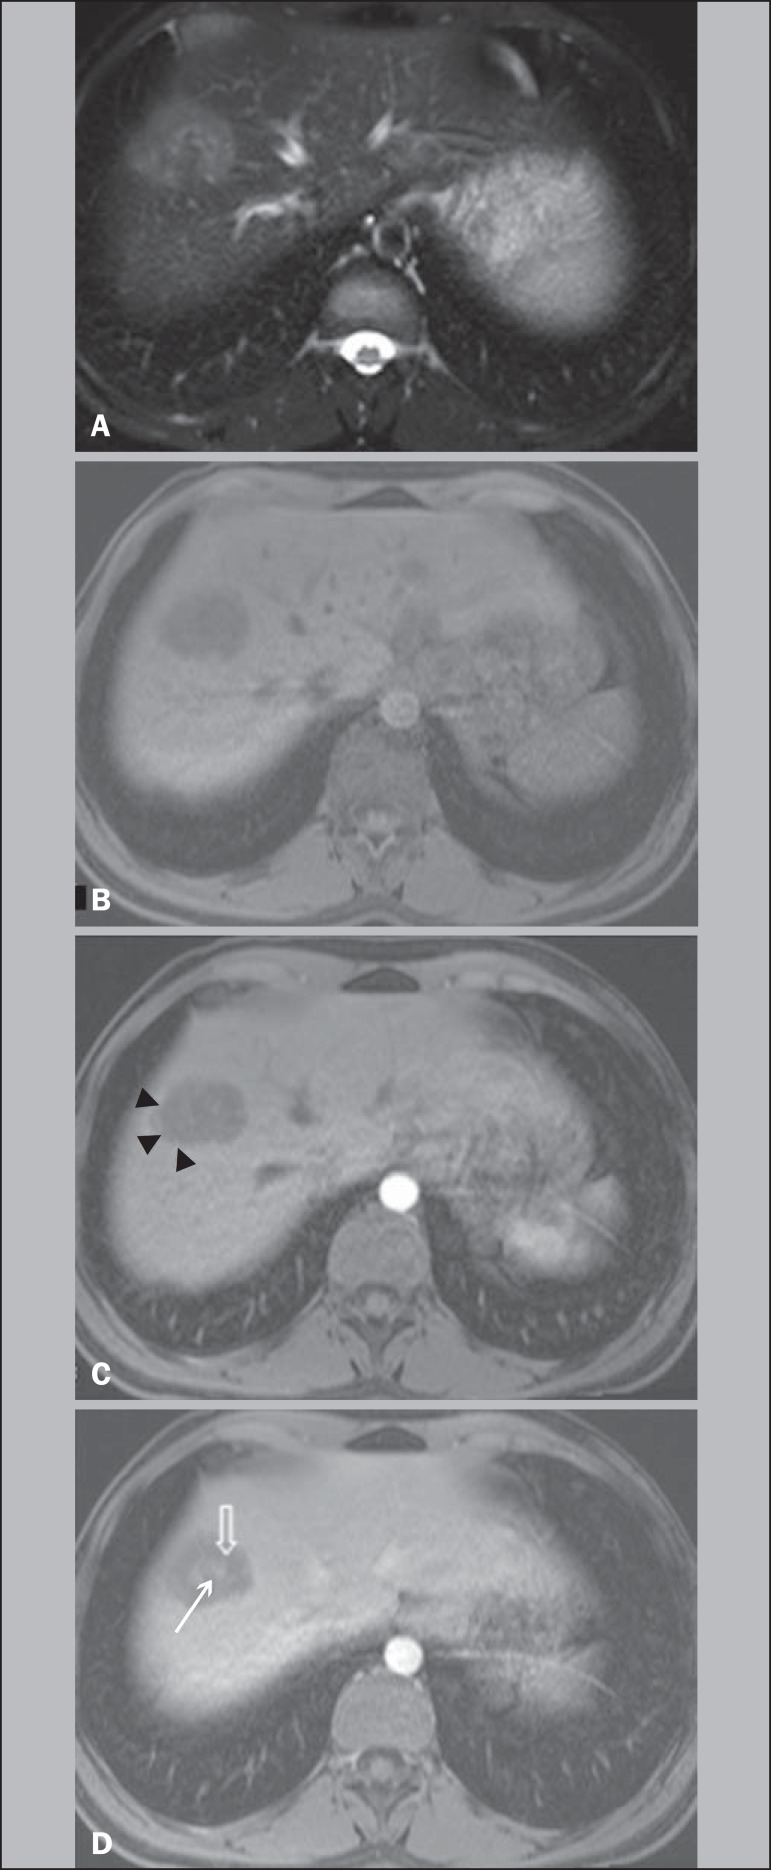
A 21-year-old female with HEHE. MR images at 1.5 T. Axial T2-weighted
